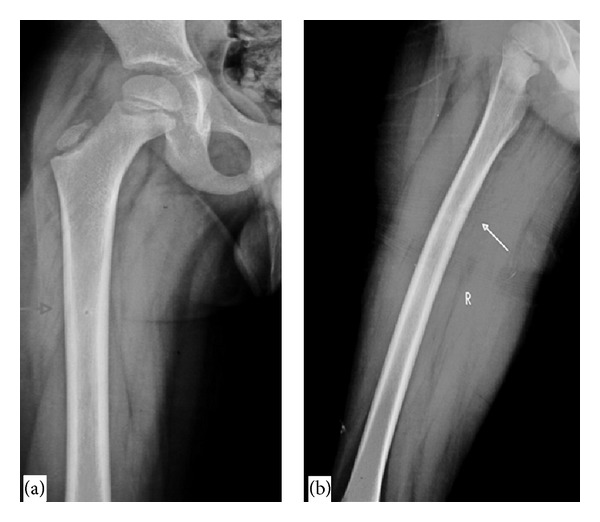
AP (a) and lateral (b) radiograph of the right femur show a well-defined linear radiolucent defect along the medial cortex of femur in its upper and middle third junction with sclerotic rim.
